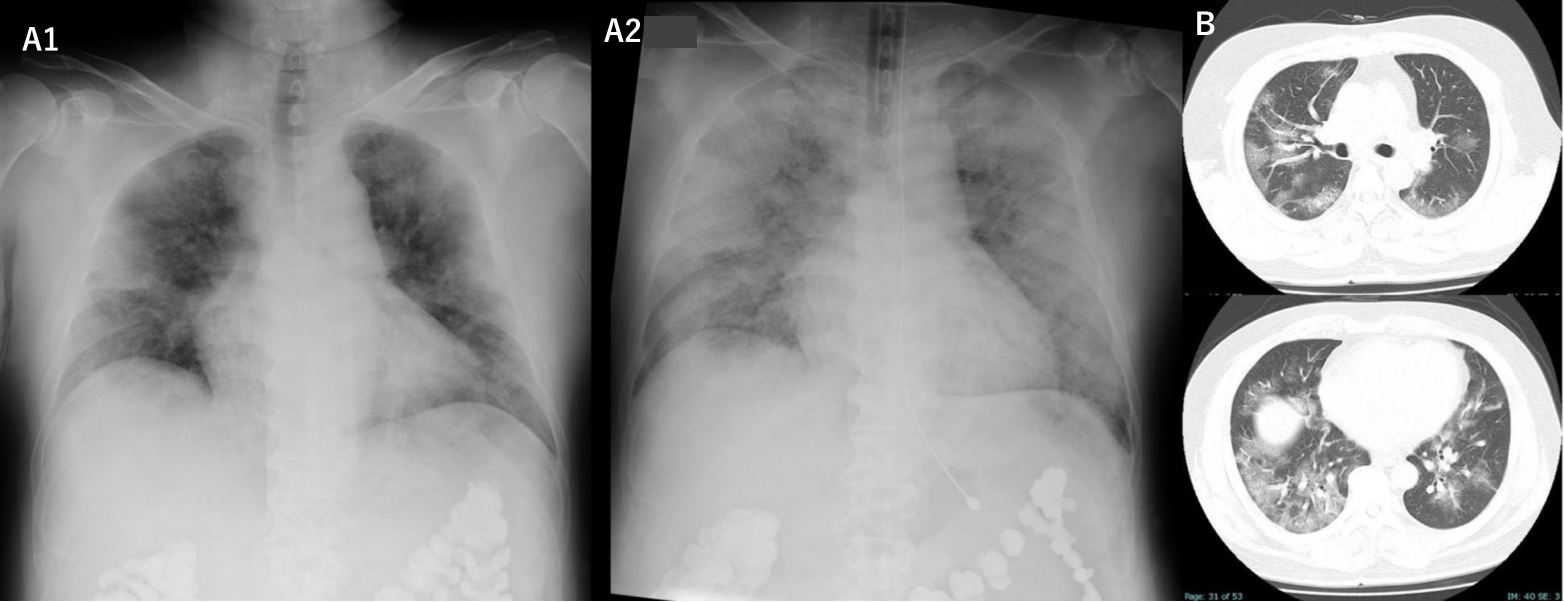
Axial images of HRCT and posteroanterior chest radiographs of patient 1. A, Chest radiograph obtained on hospital day2, before intubation, showed extensive interstitial shadows in both lungs (A1). Follow up radiograph after intubation revealed drastically increased opacities (A2). B, CT obtained on hospital day2, before intubation, showing widely spread of ground-glass opacities with crazy-paving appearance in both lungs
